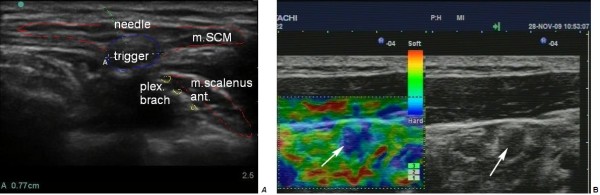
Transverse scan and the trigger point. (A) Transverse scan of the jugular region. Ultrasound visualization of the trigger points (trigger) and the needle inserted under ultrasound guidance. plex. brach, brachial plexus; m.SCM, sternocleidomastoid muscle; m. scalenus ant., anterior scalenus muscle. (B) The trigger point in anterior scalene muscle. Sonoelastography application depicts the blue area of rigid muscle tissue (arrows).
